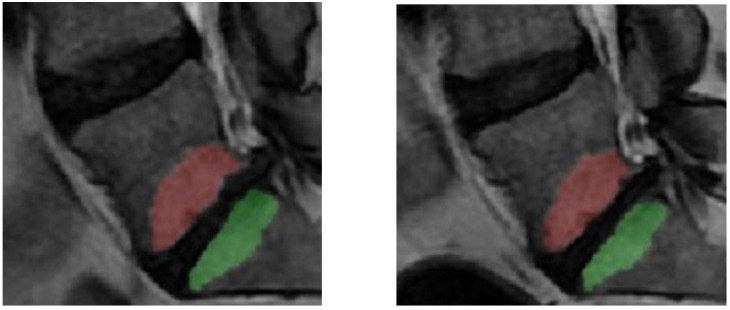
Example of segmented Modic change (MC) in L5 and in S1 at MRI without (left) and with (right) spinal loading.
